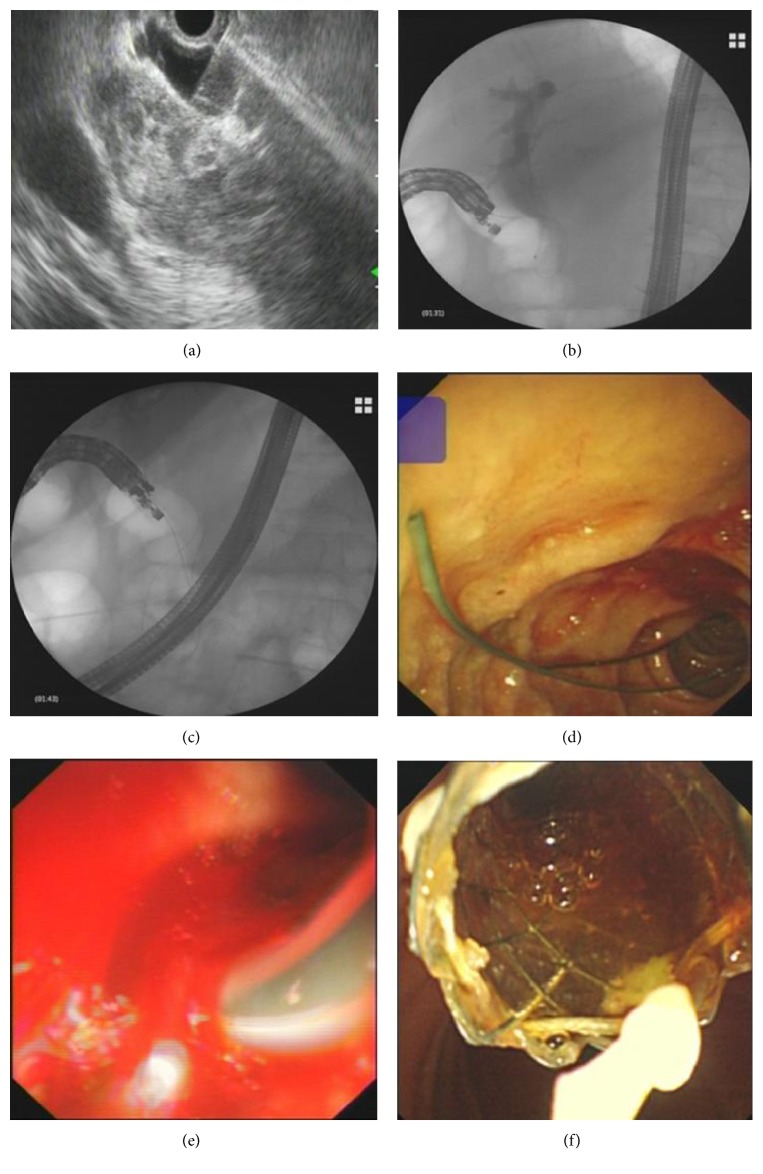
Endoscopic ultrasound-guided rendezvous technique. Under real-time EUS guidance, the dilated bile duct was punctured and a cholangiogram was obtained (a and b). A guidewire was advanced in an antegrade direction through the site of stenosis and across the papilla (c and d). Biliary cannulation was performed in the usual manner over the guidewire (e). Finally, an uncovered self-expandable metal stent was deployed for biliary drainage (f).
